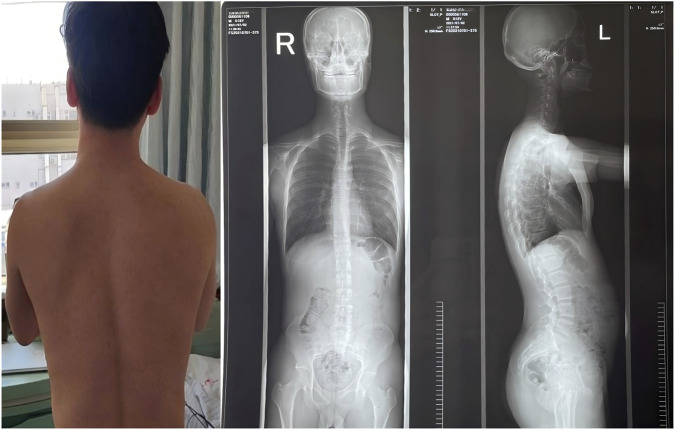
Full-length standing anteroposterior and lateral X-ray images of the spine. The thoracic spine exhibits a mild rightward curvature centered at T7, while the lumbar spine demonstrates a mild leftward curvature centered at L3. No obvious abnormalities were observed in the cervical and sacrococcygeal spine.
